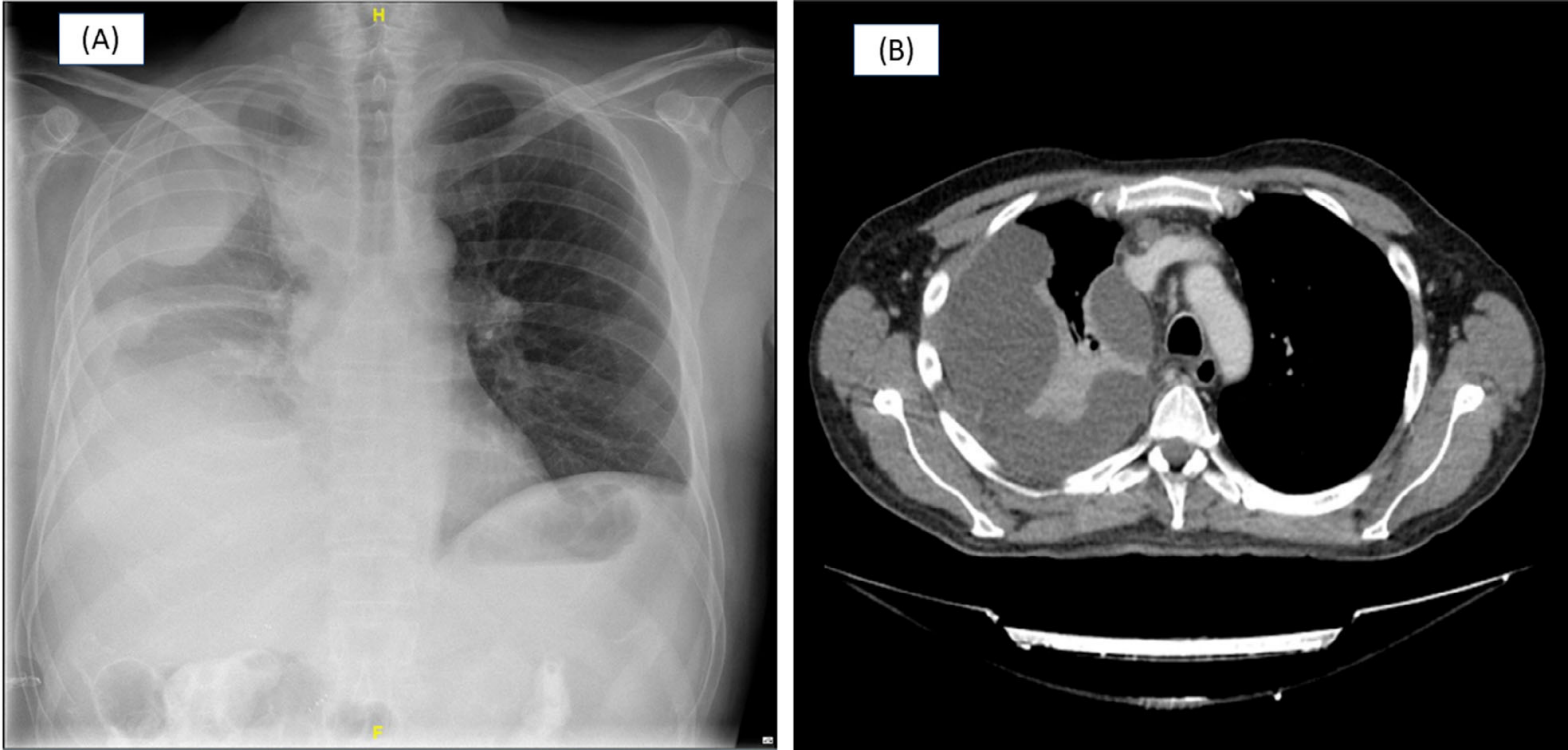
(A) Anteroposterior chest radiograph showing significant right loculated pleural effusion. (B) Computed axial tomography image of the chest showing significant right pleural effusion of loculated appearance occupying most of the right hemithorax except for a part of the pulmonary apex and middle lobe, with passive atelectasis of the adjacent lung parenchyma.
